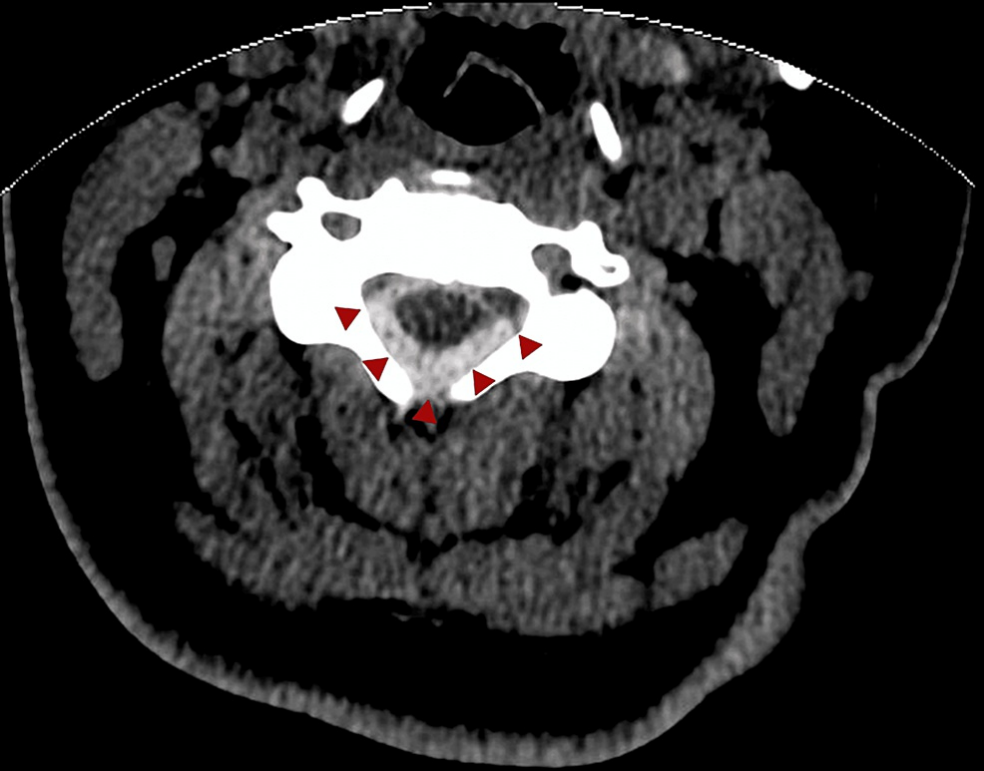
Axial non-contrast computed tomography image of cervical spine demonstrating subarachnoid hemorrhage
